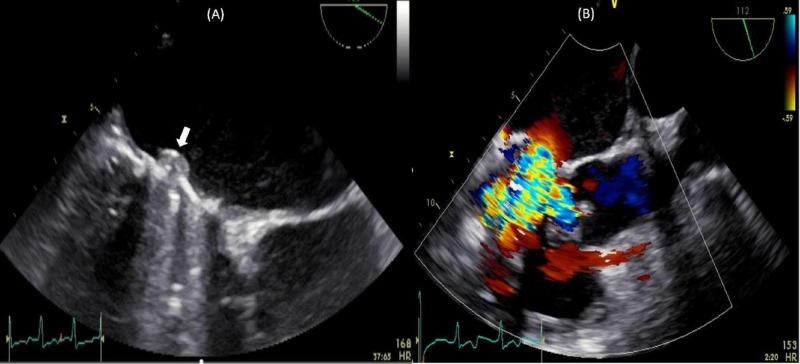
Trans-esophageal echocardiography mitral valve focused view. Demonstrating a mass (white arrow) on the mechanical mitral valve concerning for thrombus (A) and turbulent flow with color doppler across the mitral valve (B).
